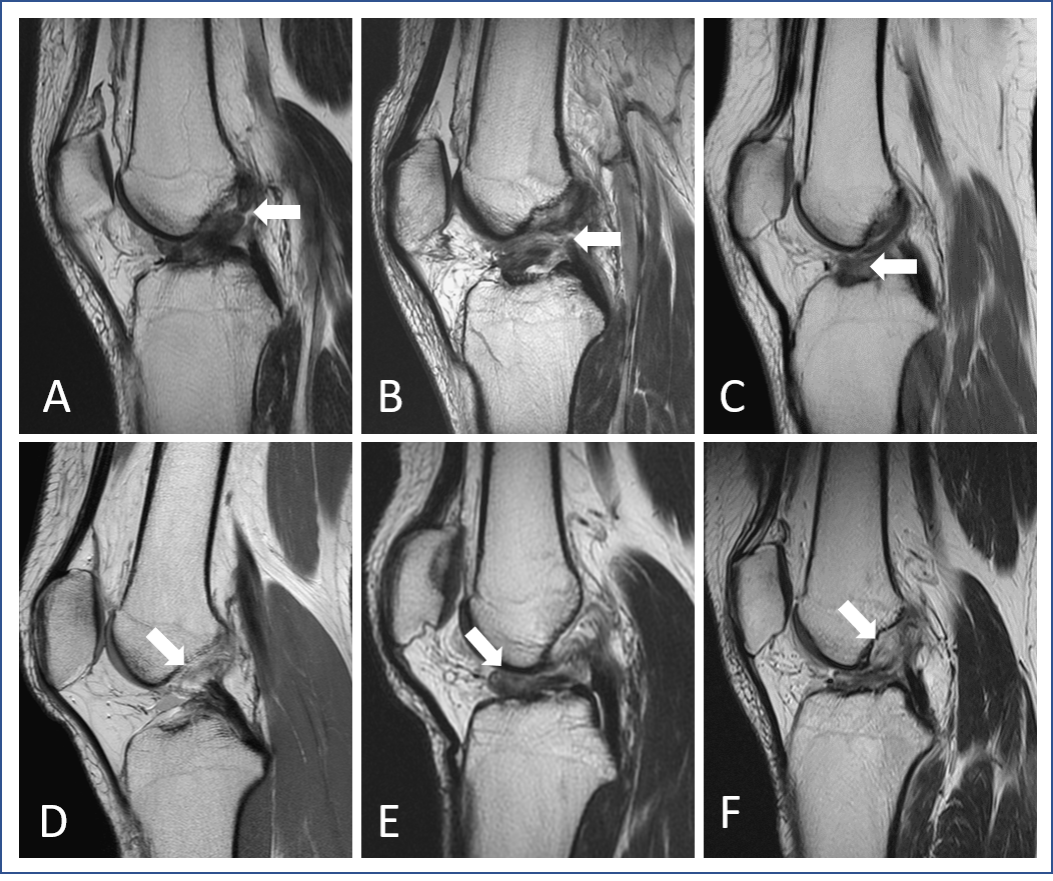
MR images of different torn-ACL patterns. Sagittal proton density images from 6 different patients show variations in the patterns of torn ACL on their respective images: (A) proximal third tear; (B) mid-substance tear; (C) distal third tear; (D) chronic tear with complete ligament resorption, such as ligament disappearance; (E) tear with folded ligament, which may cause extension difficulty; and (F) tear with cyst formation. White arrow: lesion site. ACL: anterior cruciate ligament; MR: magnetic resonance.
